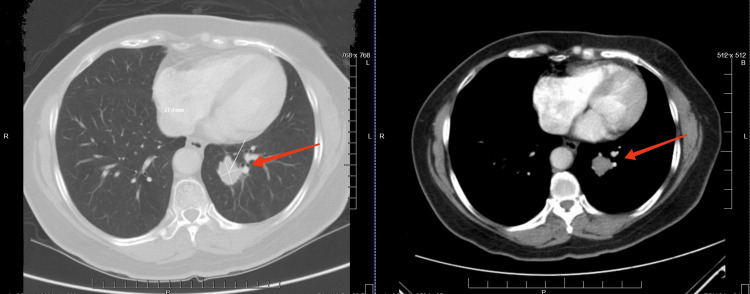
Chest CT with contrast showed a ~3 × ~3 cm nodule in the retrocardiac area in the lower lobe of the left lung.
